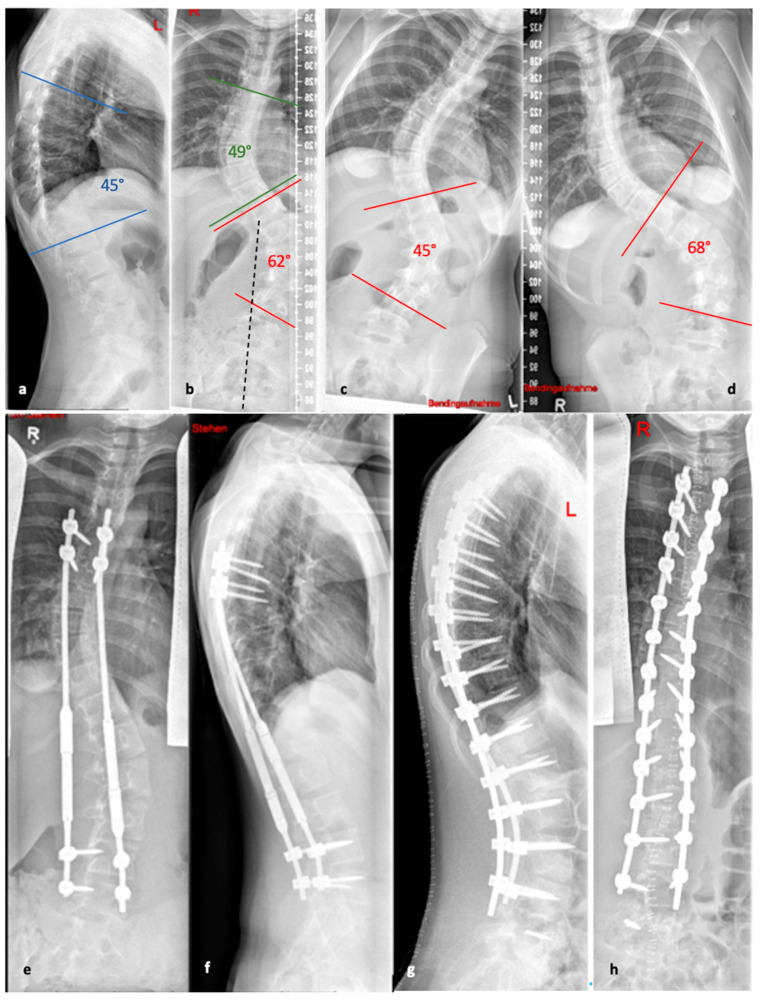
(a,b). Initial radiographs of a patient with adolescent idiopathic scoliosis. (c,d). Bending radiographs. (e,f). Postoperative image displaying implanted magnetically controlled growing rods as a dual rod system. (g,h). The patient was treated with a spondylodesis upon the completion of growth to maintain correction success (Reprinted with permission [10]).
